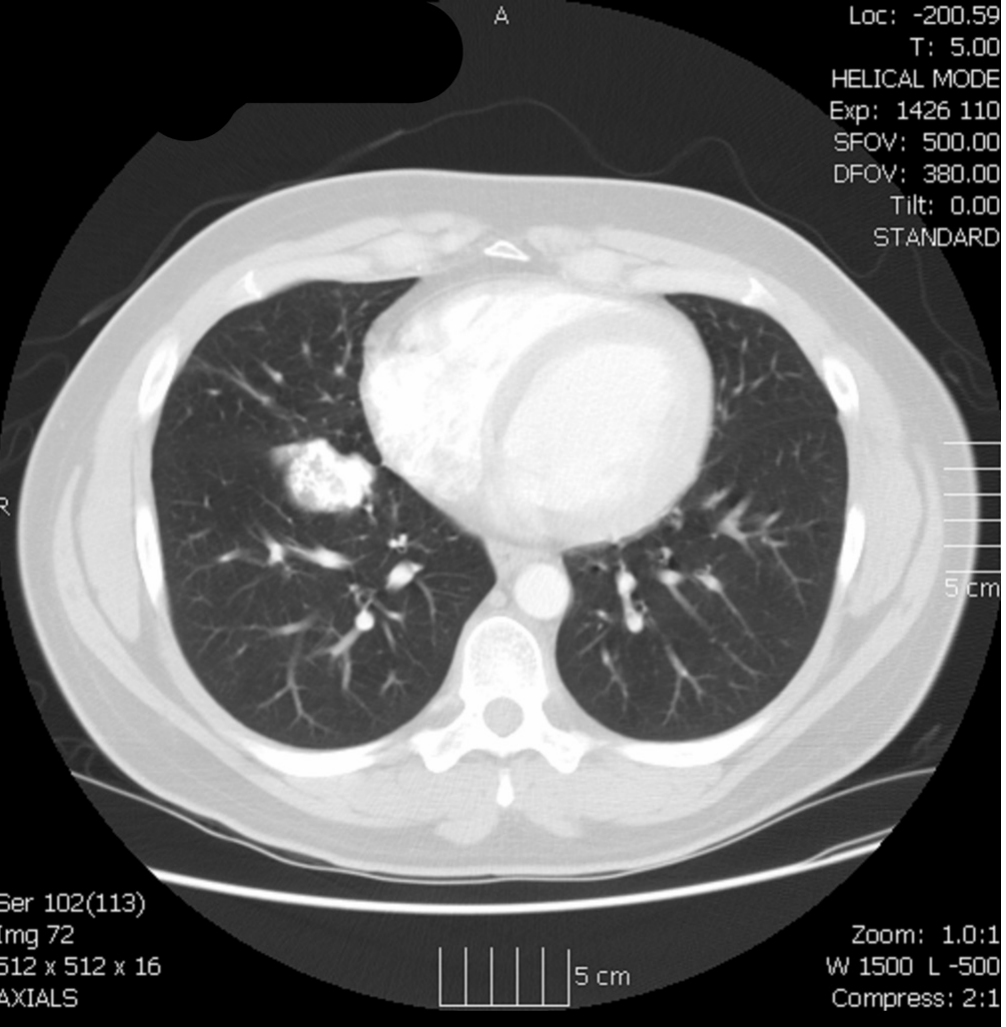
Transaxial chest computed tomography scan demonstrating a calcified tumor in the right middle lobe adjacent to the oblique fissure.
